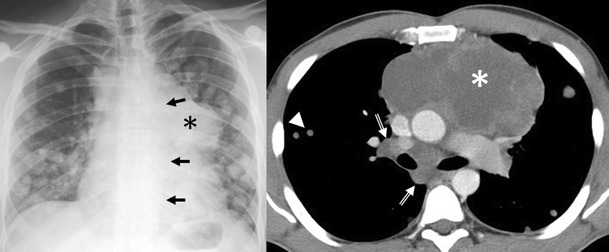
Non-seminomatous malignant germ cell tumour of the anterior mediastinum in a 25-year-old man with chest pain and high serum level of α-fetoprotein at admission (25.396 ng/ml). Frontal chest radiograph shows a central mass (*). The descending aorta is clearly seen (*arrows*), indicating that the mass is not within the posterior mediastinum. Multiple nodules in bilateral pulmonary field are also observed. A contrast-enhanced CT scan confirms a mass of low attenuation (*) in the anterior mediastinum that compresses the pulmonary artery. Bilateral lung metastasis (*arrowheads*) and hilar and subcarinal lymphadenopathy is identified (*open arrows*)
